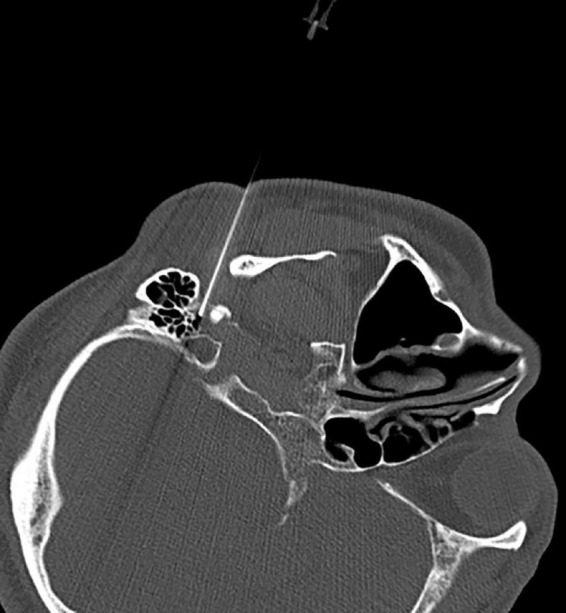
Puncturing of the stylomastoid foramen under intermittent CT guidance.
